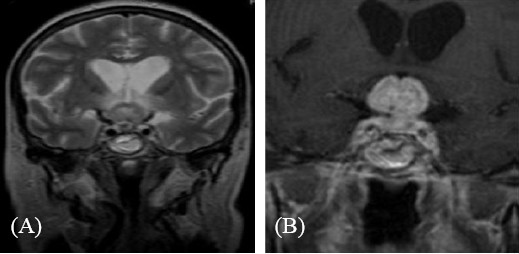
T1-weighted magnetic resonance imaging scan of the brain. (A) Coronal image of pituitary metastasis causing compression of the optic chiasm; (B) detail of optic chiasm compression.
